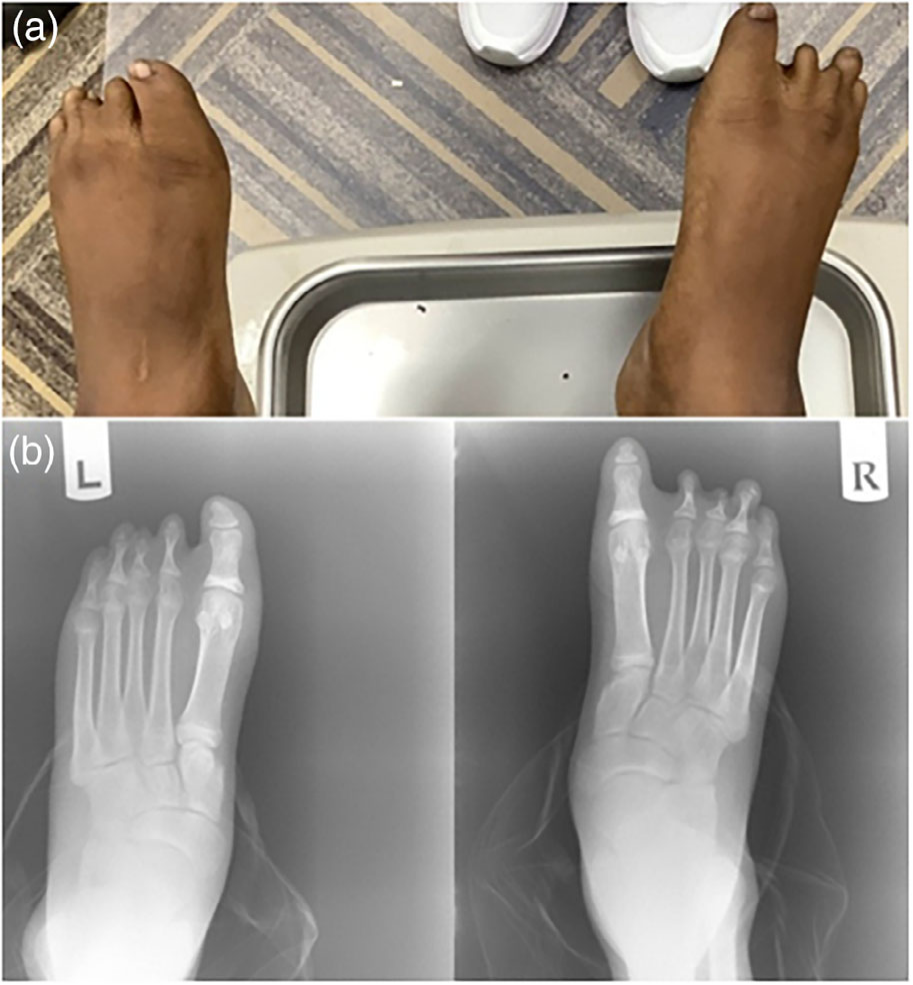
A) Photo of the boy's left and right feet at 13 years of age; digital malformations are clearly shown in the picture. B) Both foot radiographs of the boy at 13 years of age show absent or malformed phalanges.
